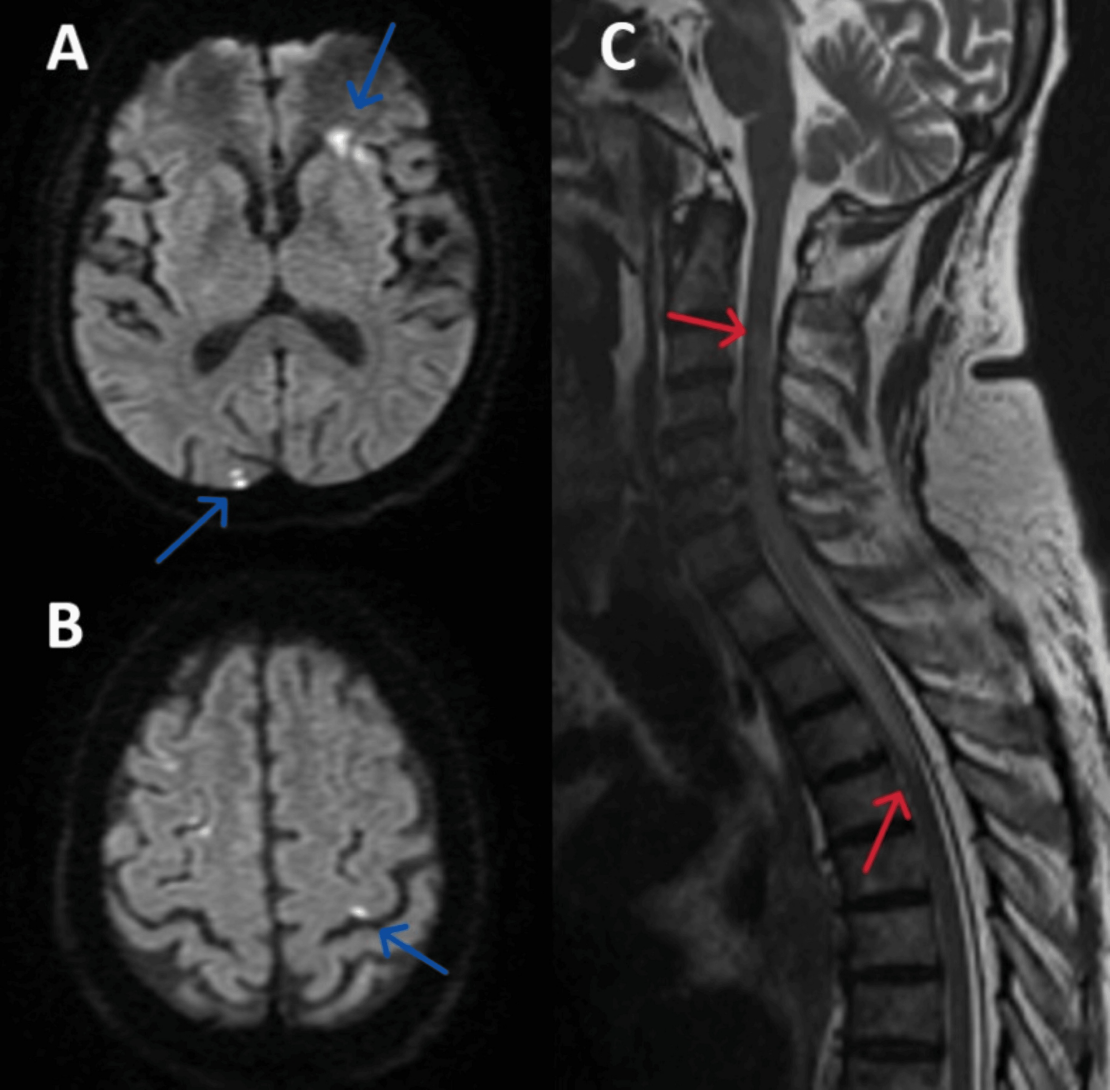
MRI findings (A, B) Areas of diffusion restrictions in bilateral cerebral hemispheres (blue arrows), (C) Long segment T2 hyperintensity in spinal cord from C3 to D3 (red arrows).
